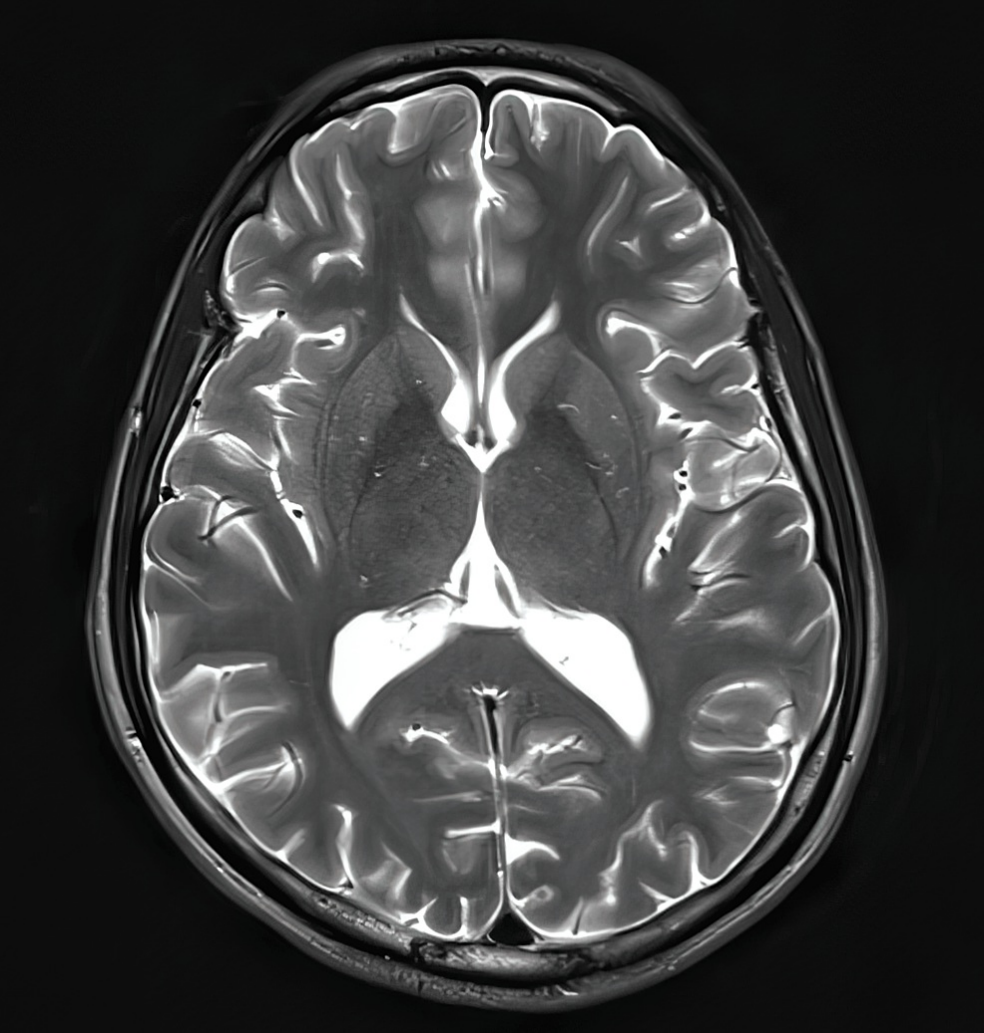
Normal MRI brain, T2 weighted image
